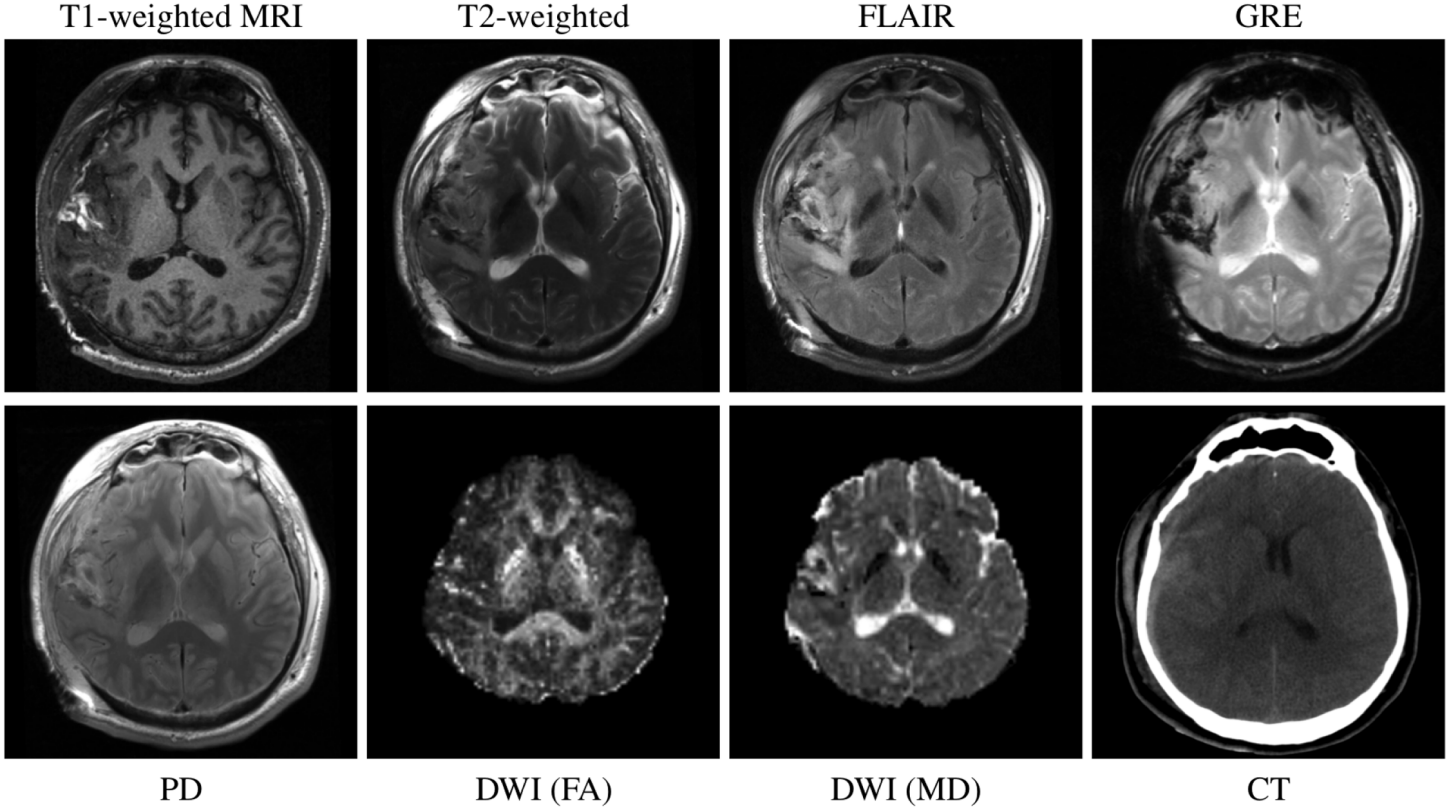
Brain images acquired with different imaging sequences/modalities. Images acquired from a patient with traumatic brain injury. The good tissue contrast in T1-weighted MR images and the pronounced contusions in the FLAIR sequence are apparent. Furthermore, gradient echo (GRE) and proton density (PD) weighted images are shown. Diffusion derived fraction anisotropy (FA) and mean diffusivity (MD) maps are also shown. CT is well suited to image bone injuries, oedema or intracranial bleeding. Note that this subject is from a different TBI dataset to the one used in this study. This study focuses on the analysis of T1-weighted images only, other sequences/modalities are shown to provide further background of MR imaging in TBI.
